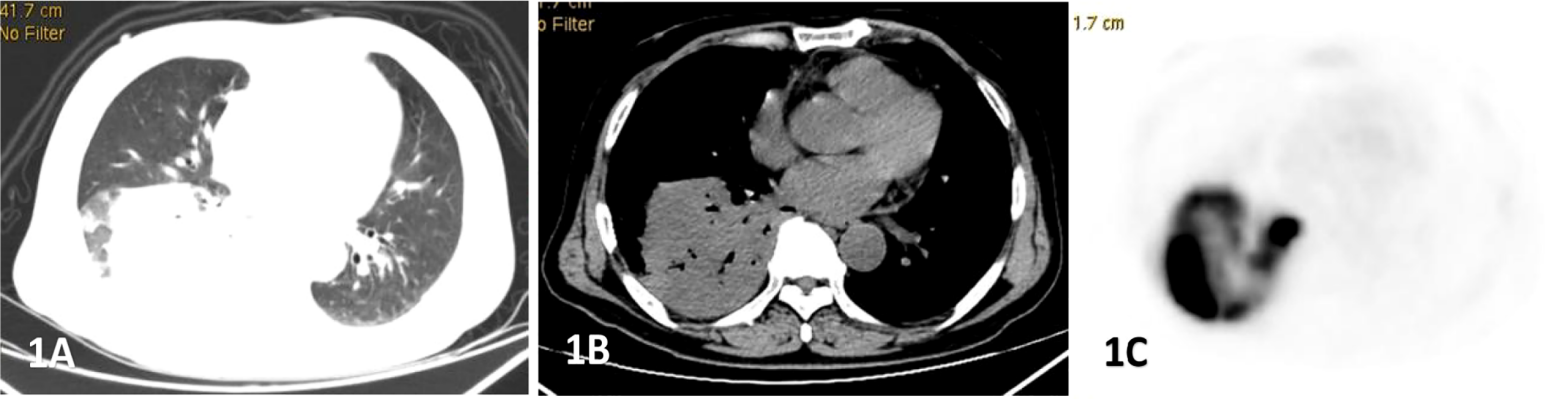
Male, 69 years old, fever, cough, dyspnea for 3 months. (A–C) showed lung, mediastinal CT scan and PET transection images respectively. Soft tissue mass shadow was observed in the lower lobe of the right lung, and aerated bronchial shadow was observed in the lesion, showing cross-lobe growth with a size of about 81×77mm.The FDG uptake of the lesion was significantly increased, with an SUVmax of 22.6. Percutaneous lung biopsy was conformed diffuse large b-cell lymphoma(DLBCL).
